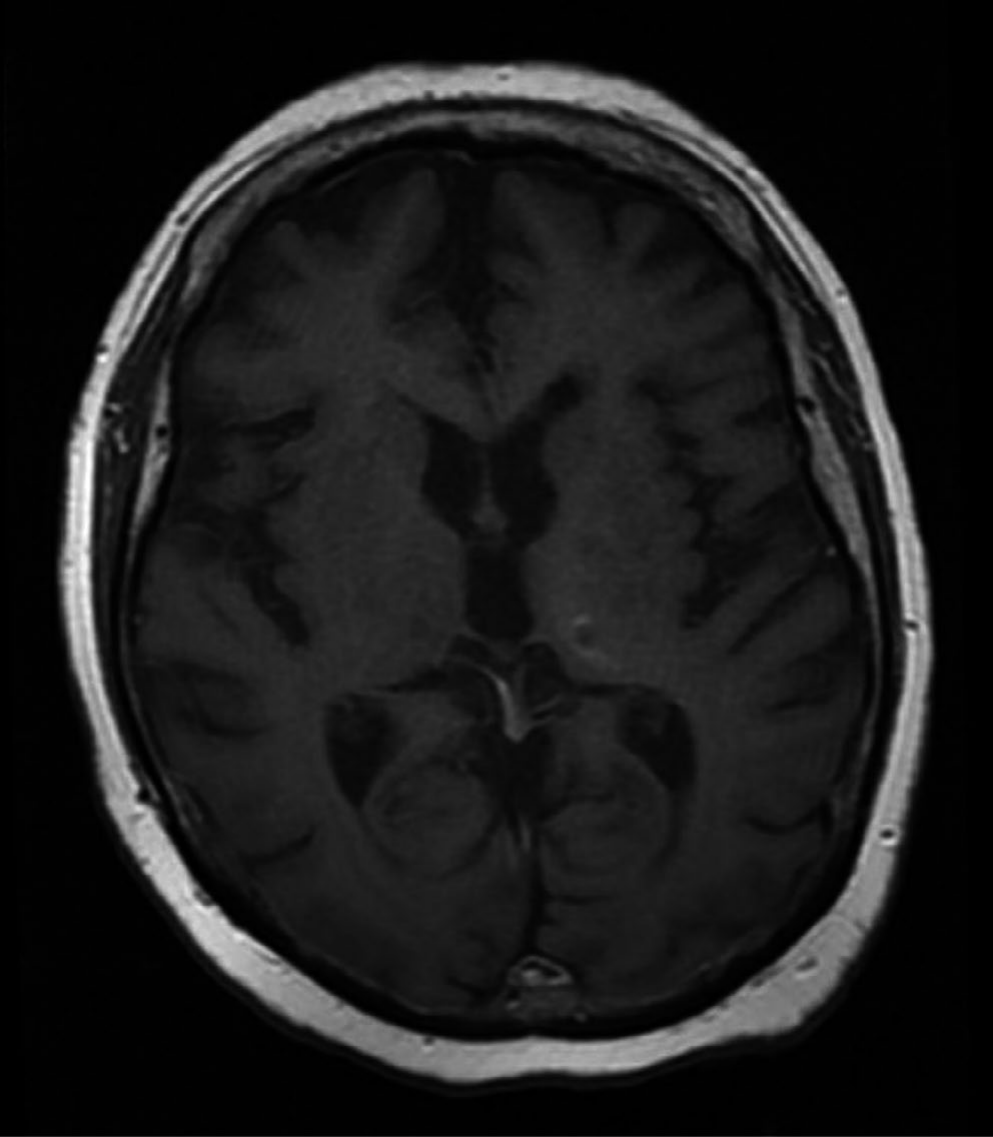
Restaging T1‐weighted magnetic resonance imaging (MRI) of the brain following 2 months of whole brain radiation therapy (WBRT) to 30.6 Gy while continuing systemic treatment with ibrutinib for diagnosed Bing‐Neel Syndrome
